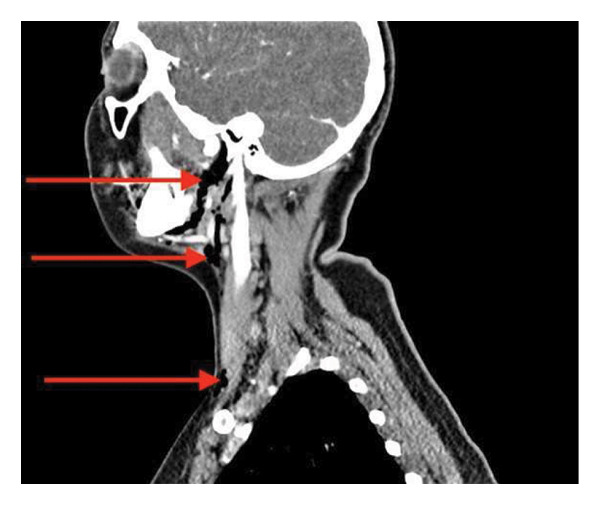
Sagittal view of a CT neck, in soft tissue window, showing surgical emphysema (red arrows) situated in the oropharynx, below the angle of the mandible and extending inferiorly to the supraclavicular space.
